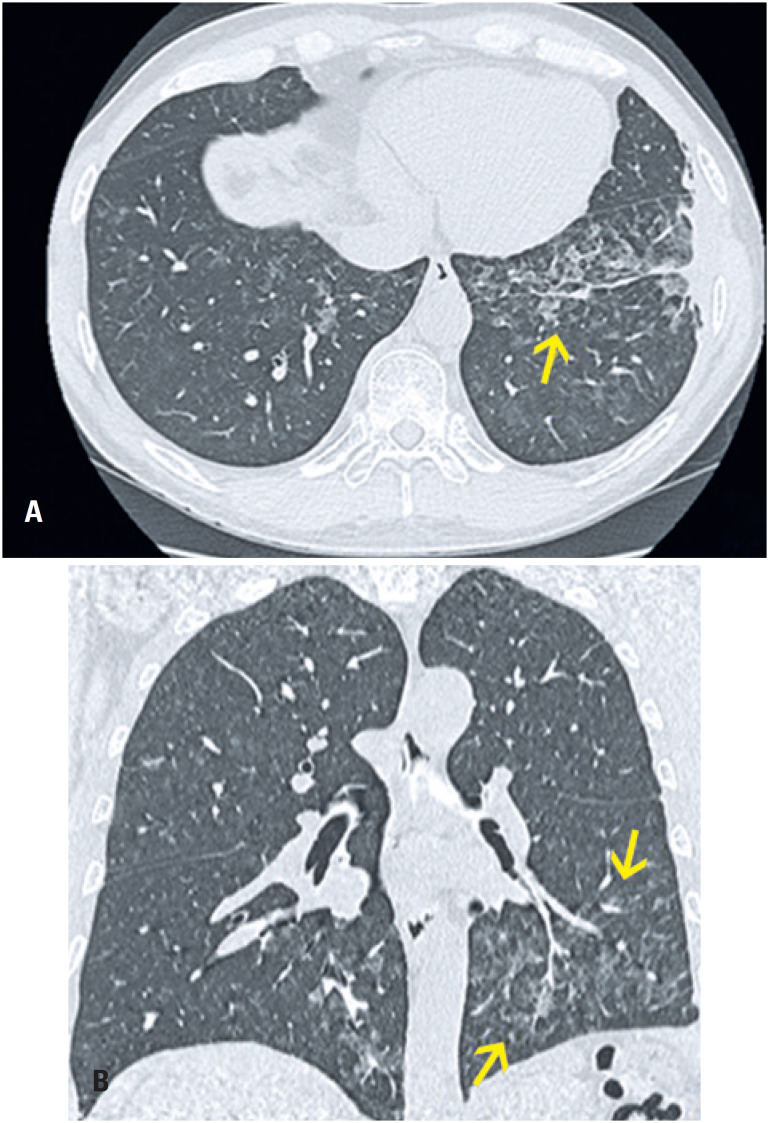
A 50-year-old asymptomatic man had the test for a medical check-up. Computed tomography (A) Axial section and (B) Coronal reformation image: multiple alveolar ground-glass opacities in both lungs, predominantly in the lower centrilobular fields. The findings were maintained in the 3-month control examination, after a biopsy was performed. The definitive diagnosis was lipoid pneumonia
